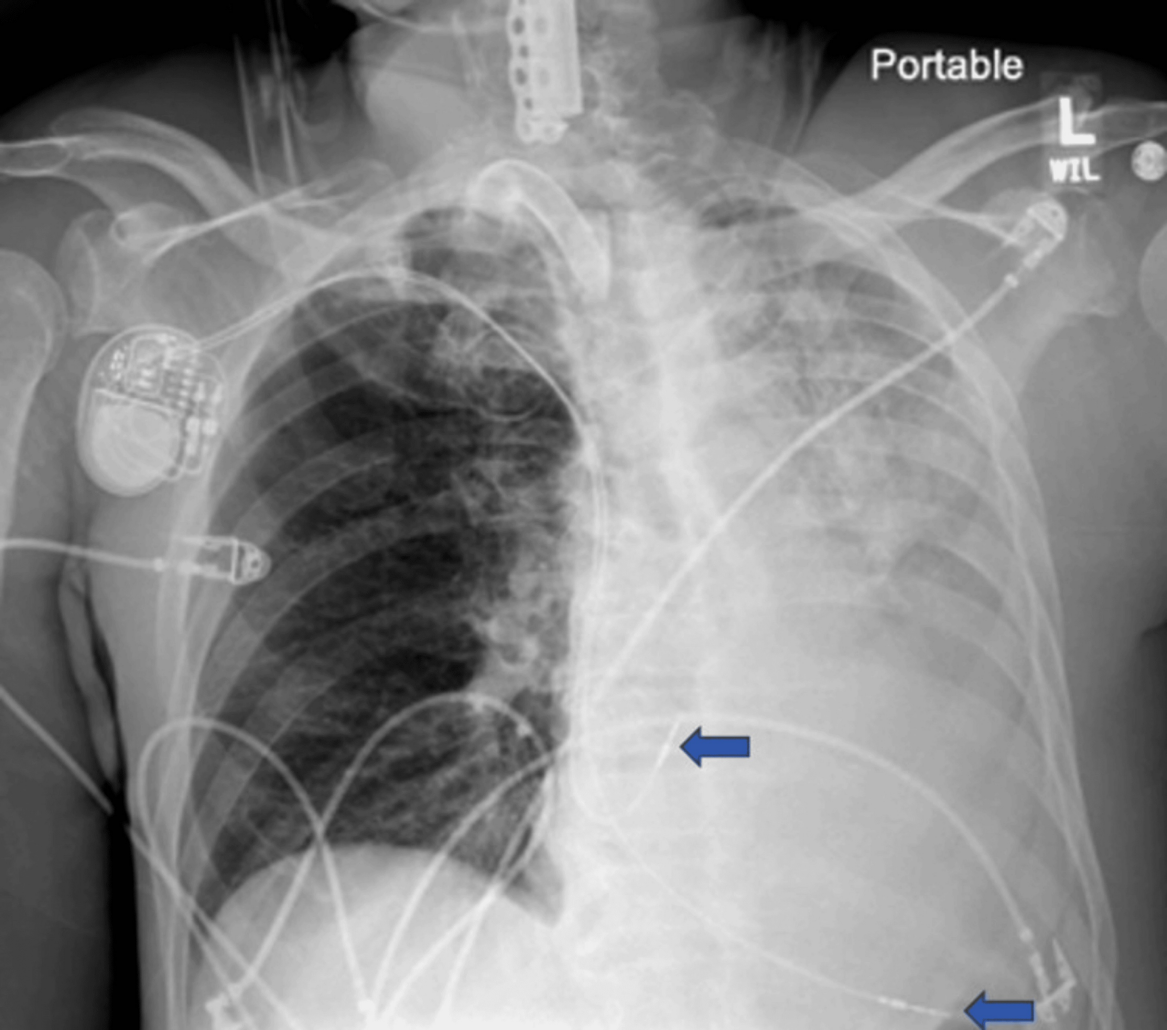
Chest X-ray revealing complete left lung whiteout with a leftward shift of the pacemaker leads (blue arrows).
